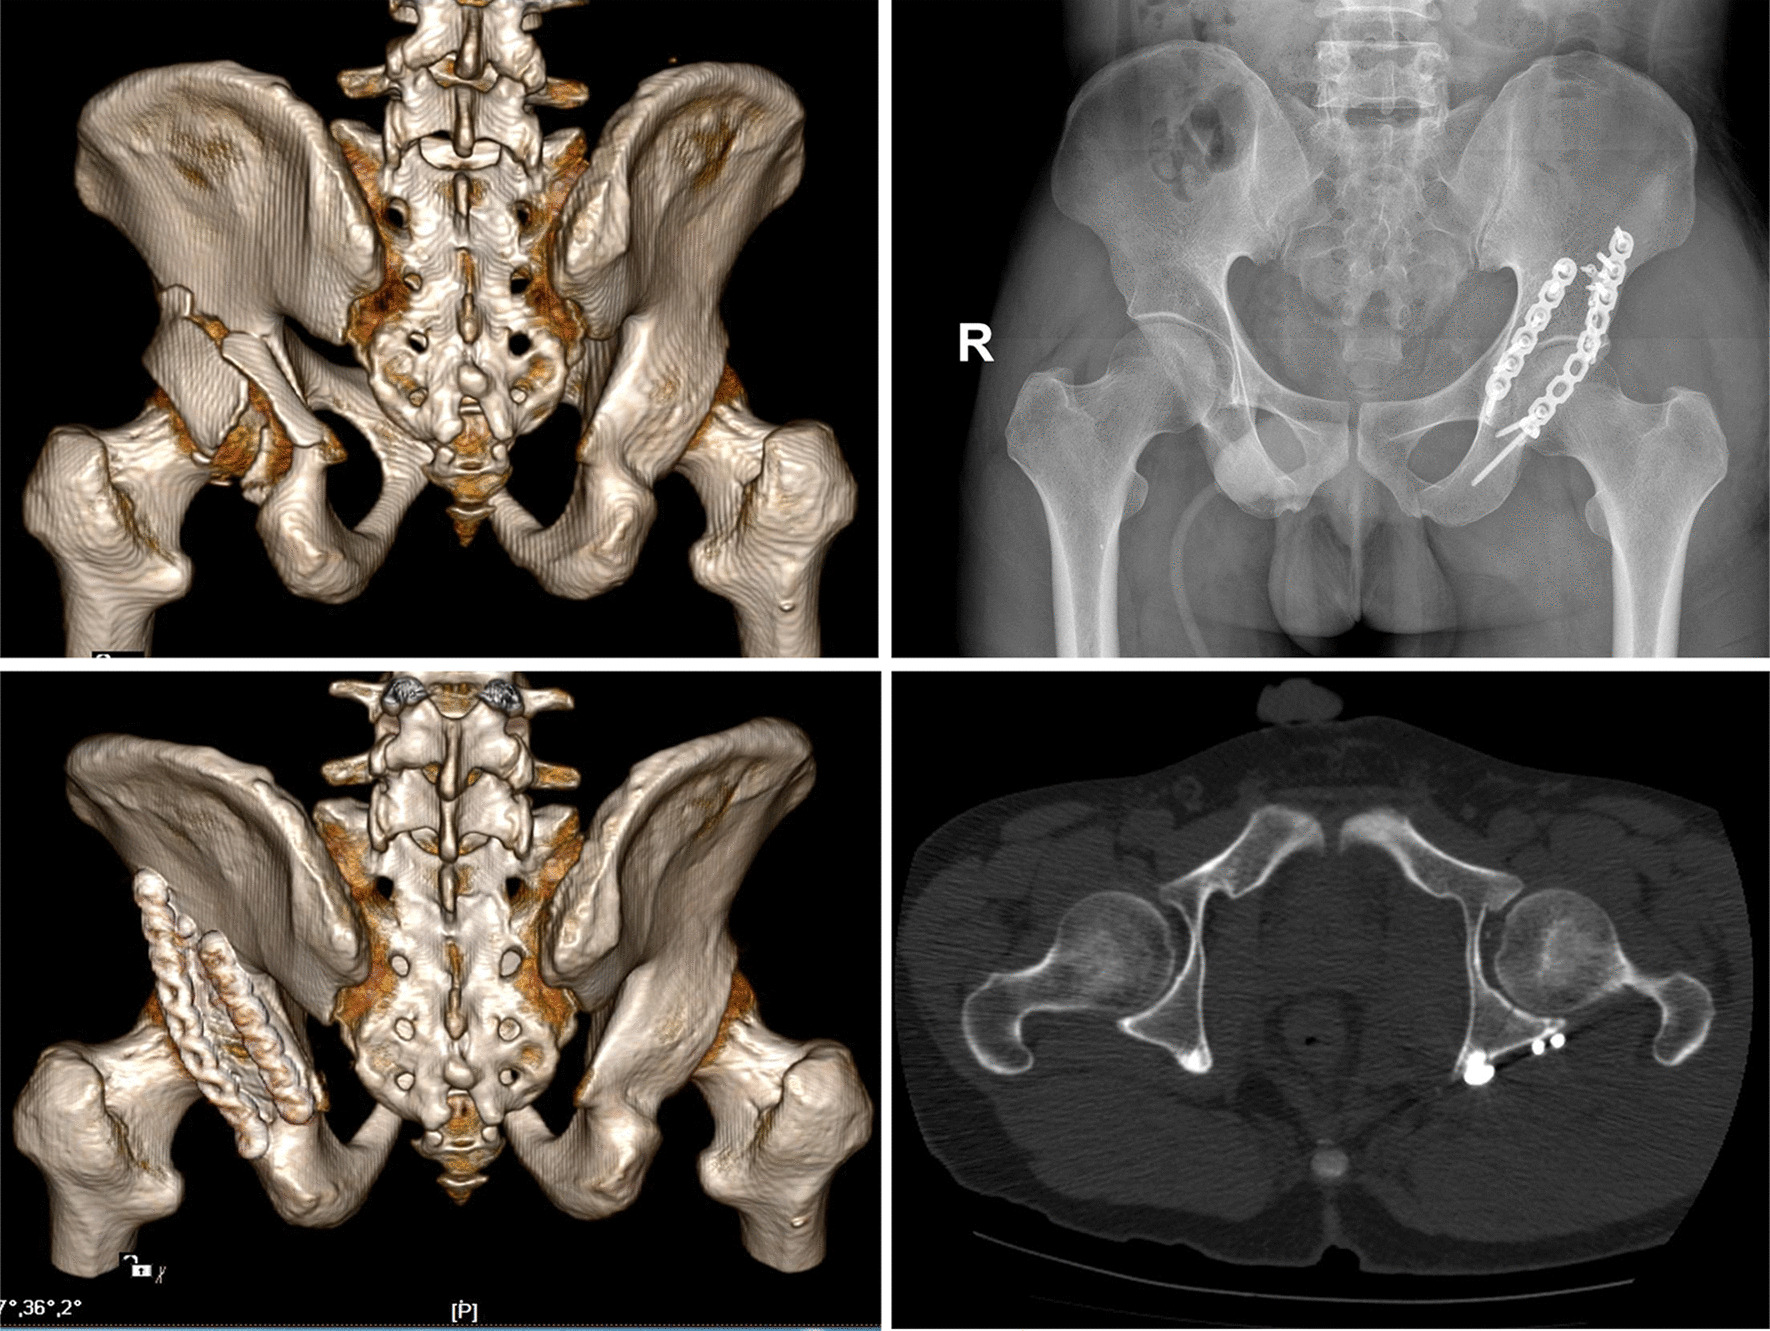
The outcomes of virtual preoperative planning were identical to the actual internal fixation mode and the cross-sectional CT image showed an anatomical reduction according to the Matta grading score
